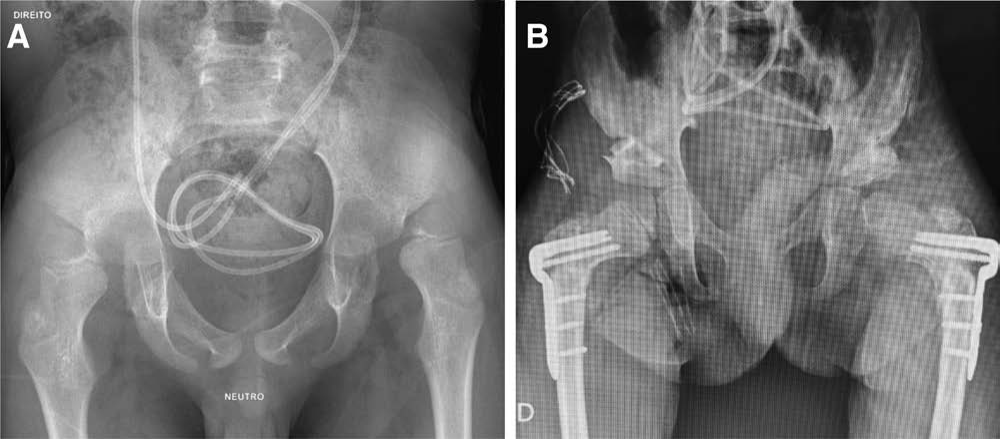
Preoperative (A) and postoperative (B) radiographs of an 8-yr-old GMFCS V boy who was submitted to a bilateral varus derotational osteotomy and a pelvic Dega osteotomy on both sides. Bilateral hip clinical stability was obtained after a closed reduction on both sides. Final Reimers Migration Percentage was 0% bilaterally. (Images from the personal files of the authors). GMFCS = gross motor function classification system.
